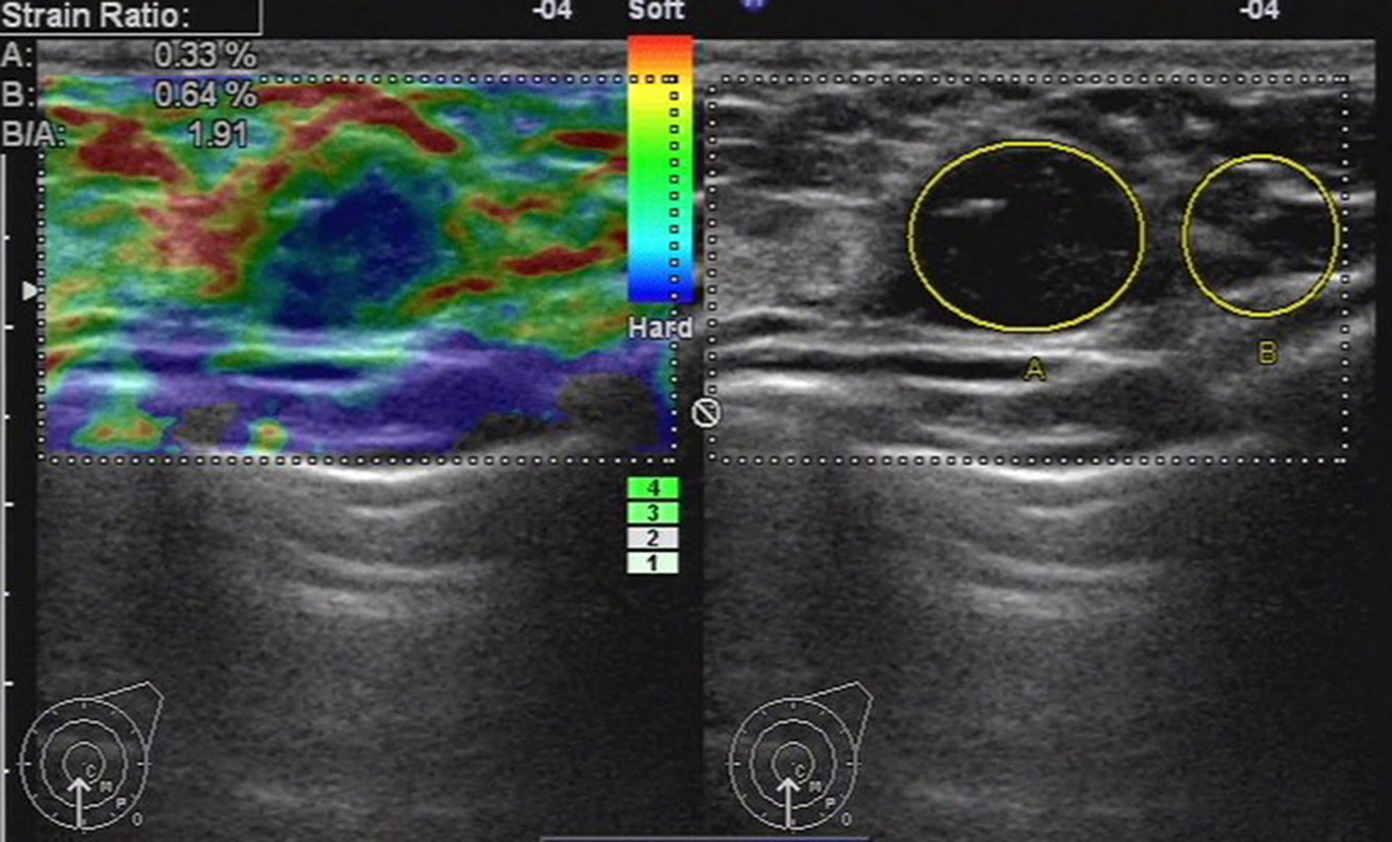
Ultrasonic elastographic image of a papiloma in a 34-year-old woman. The lesion was scored 3 with the 5-point scoring method. The strain ratio was 1.91 with the strain ratio method at the same breast tissue depth as the reference.
